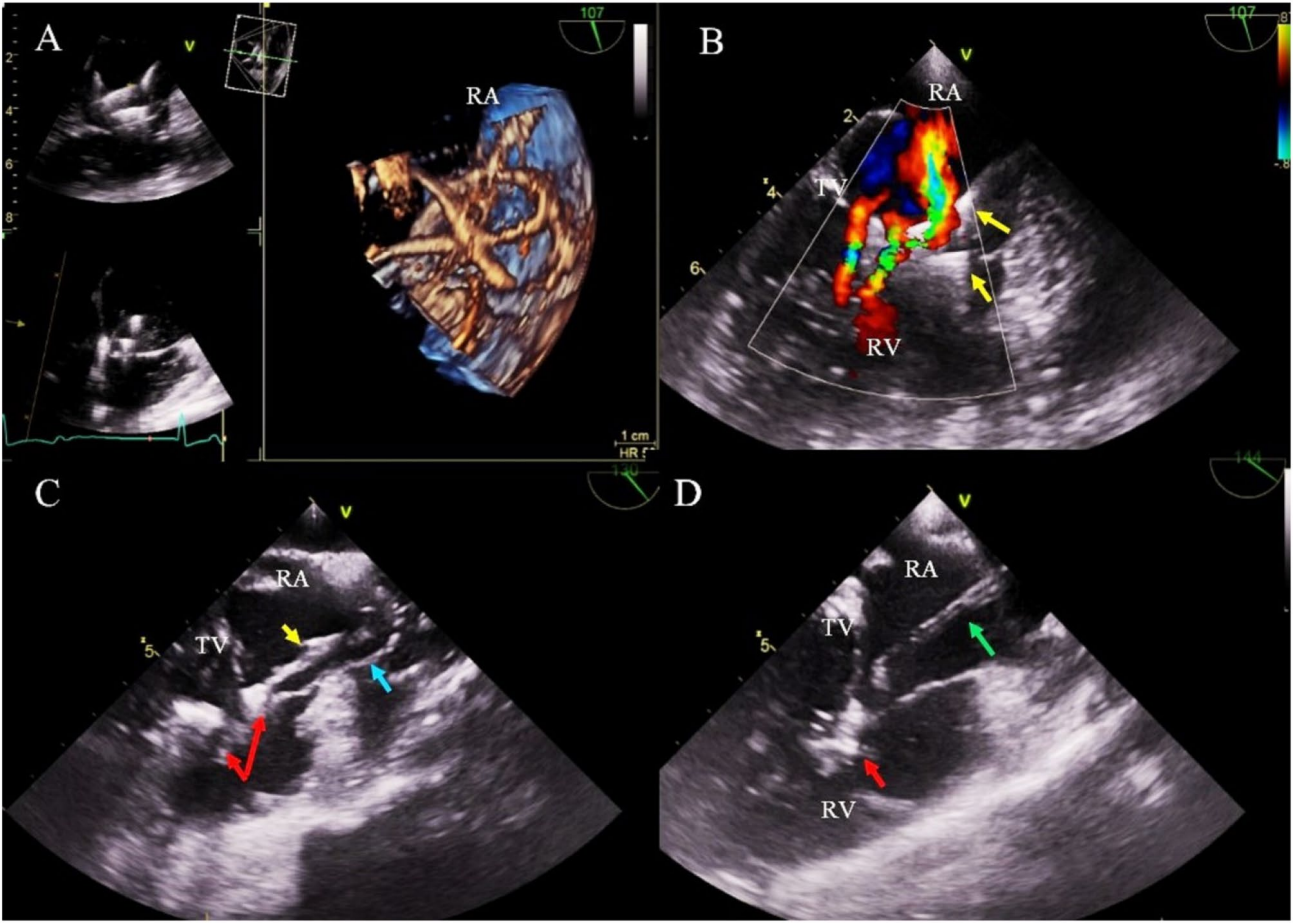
TEE images from the monitoring of the extraction of 4 leads in a 24-year-old female patient. (A) In the right atrium, loops of 4 leads fused together, displaced and adhered to the tricuspid apparatus. (B) Color Doppler—multi-flux, moderate tricuspid regurgitation with moderate valve stenosis (V max 1.6 m/s, PG avg. 4 mmHg) resulting from conglomerate of the leads (yellow arrows). (C) Moment of extraction of the lead; one of the ventricular leads is torn and stretched (blue arrow). Pulled up the second of the ventricular leads (yellow arrow) with simultaneous pull-up of the right ventricular wall and elements of the tricuspid apparatus. Red arrows mark massive adhesions of the leads with RV and TV structures. (D) After extraction of the leads, in the RA, a fragment of the silicone insulation was visualized by TEE examination (green arrow). Massive fragments of connective tissue within the sub-valvular apparatus (red arrow), hindering the proper mobility of the valve leaflets.
